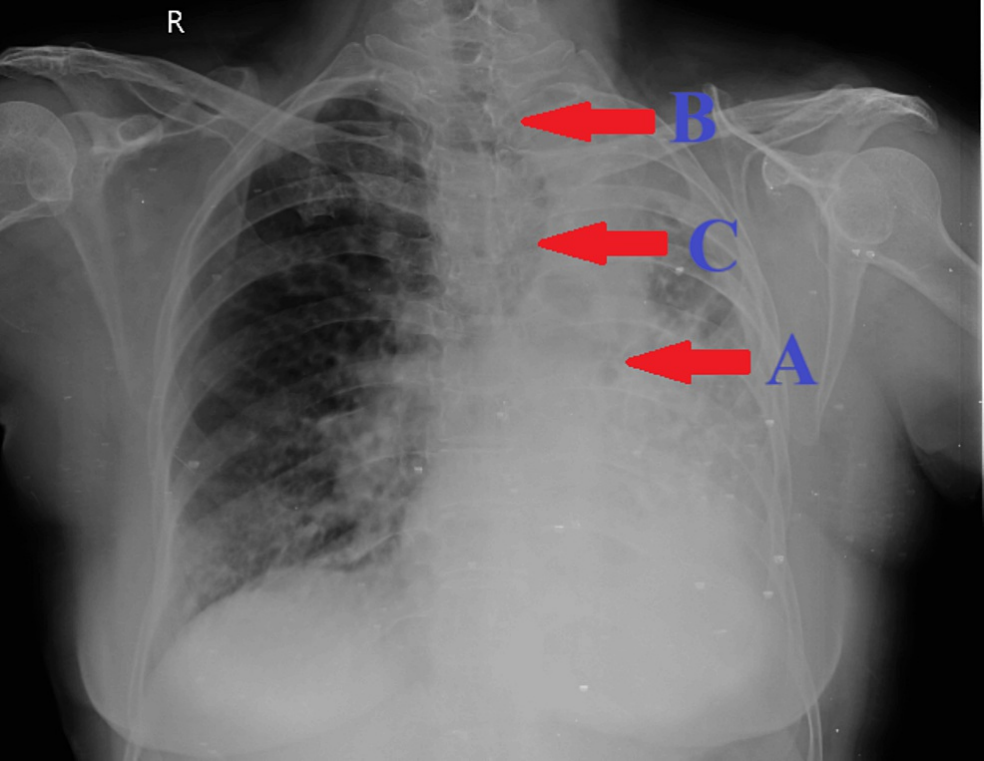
A chest X-ray in PA view displaying (A) extensive destruction of the parenchyma of the left lung; (B) tracheal and mediastinal shift toward the diseased side; and (C) herniation of a portion of the right lung into the left hemithorax PA, posteroanterior
